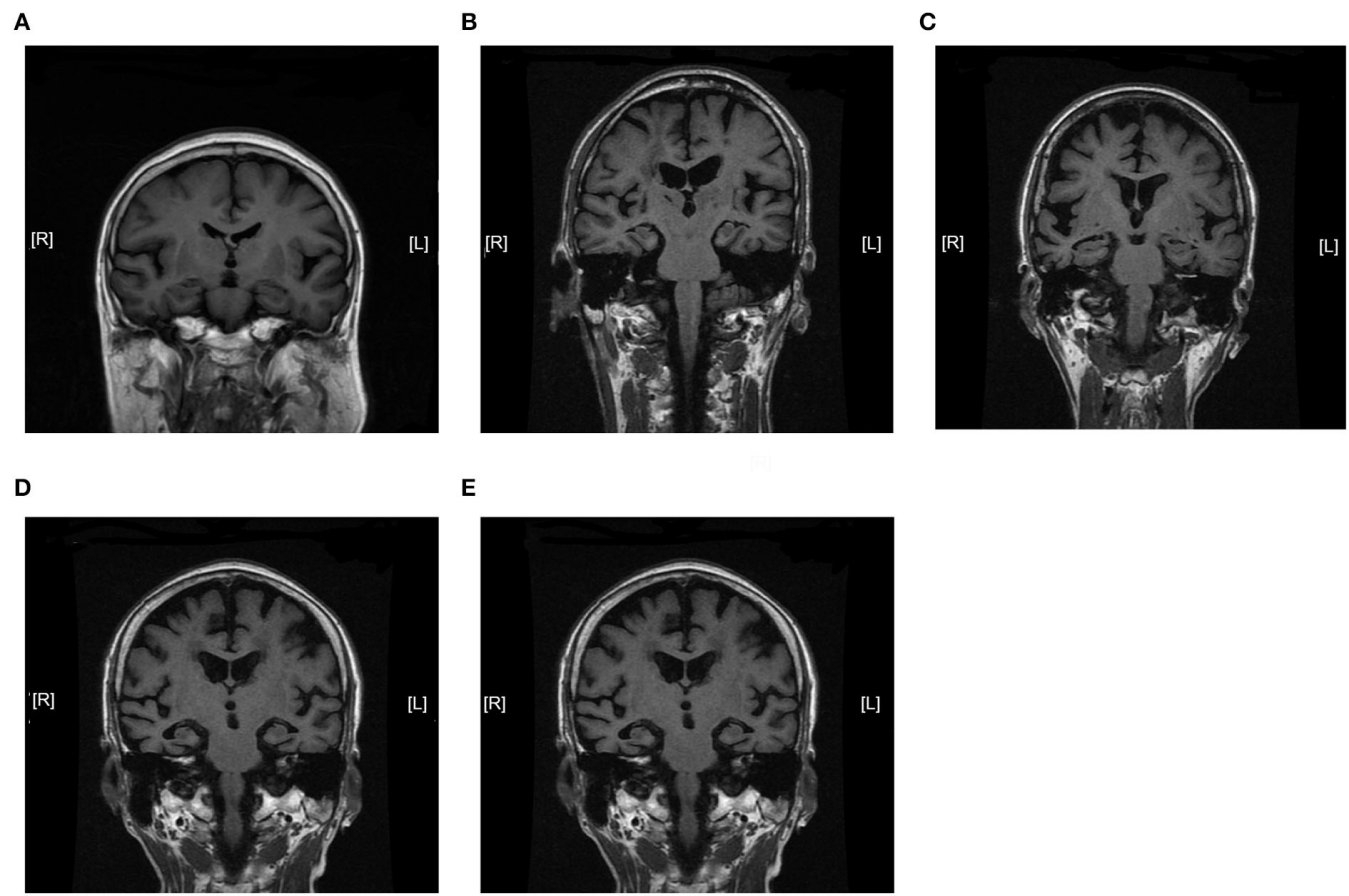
Magnetic resonance imaging coronal view of the hippocampus in T1-weighted images. MTA scores were used to assess AD progression. The degree of MTA correlates with AD severity. (A) MTA = 0. (B) MTA = 1. (C) MTA = 2. (D) MTA = 3. (E) MTA = 4. MTA: medial temporal lobe atrophy scale. MTA scores range from 0 to 4.
